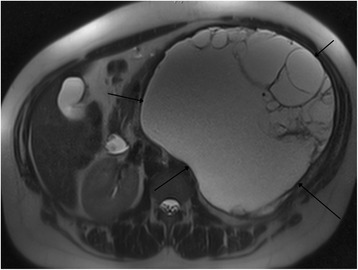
Magnetic resonance imaging of the abdomen showing large complex cyst between the *black arrows*
